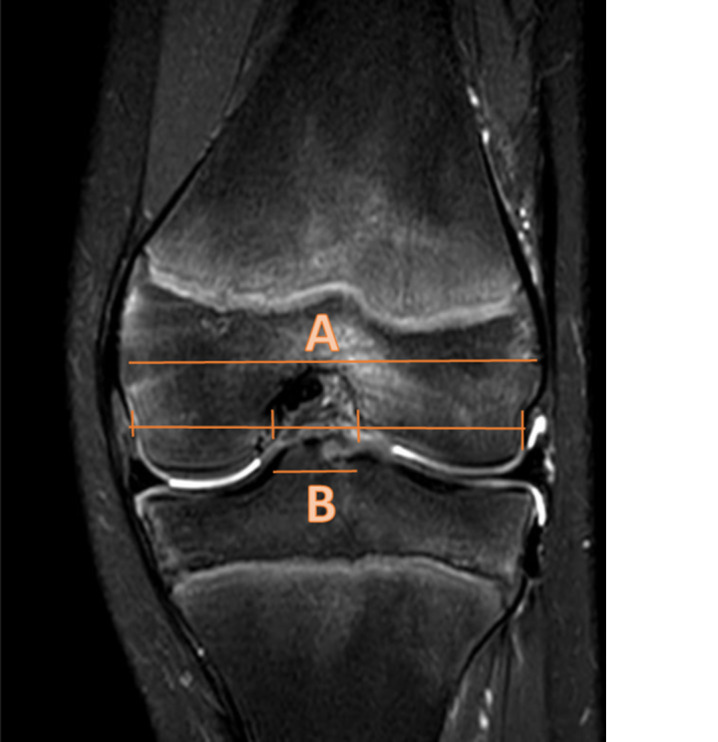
Notch width index (NWI). The NWI is measured on MRI by measuring the bicondylar width at the level of the popliteal groove in the lateral condyle of the femur, parallel to the joint line as formed by the distal femoral condyles (A). Then the most inferior margins of the intercondylar notch are identified. The distance between these points represents the intercondylar notch width (B). The NWI represents the ratio of the intercondylar notch width to the bicondylar width (B/A) [55]. MRI, magnetic resonance imaging.
